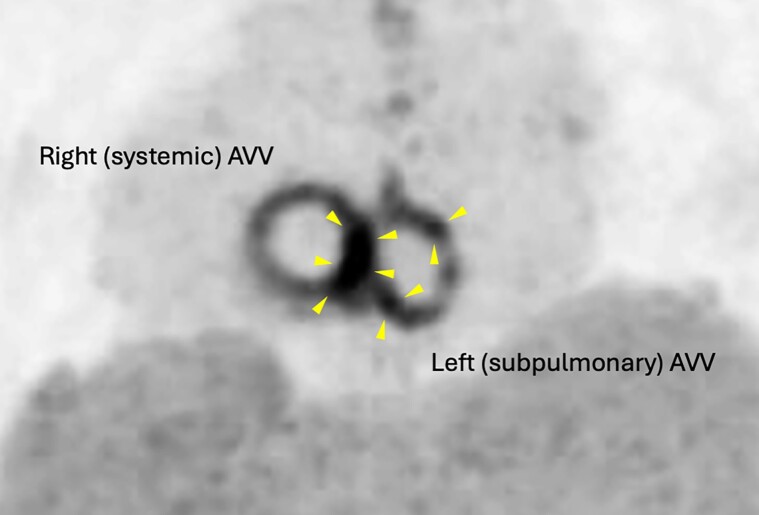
^18^F-Fuorodeoxyglucose positron emission tomography/computed tomography showing ^18^F-ﬂuorodeoxyglucose uptake with nodules (arrowheads). AVV, atrioventricular valve.
